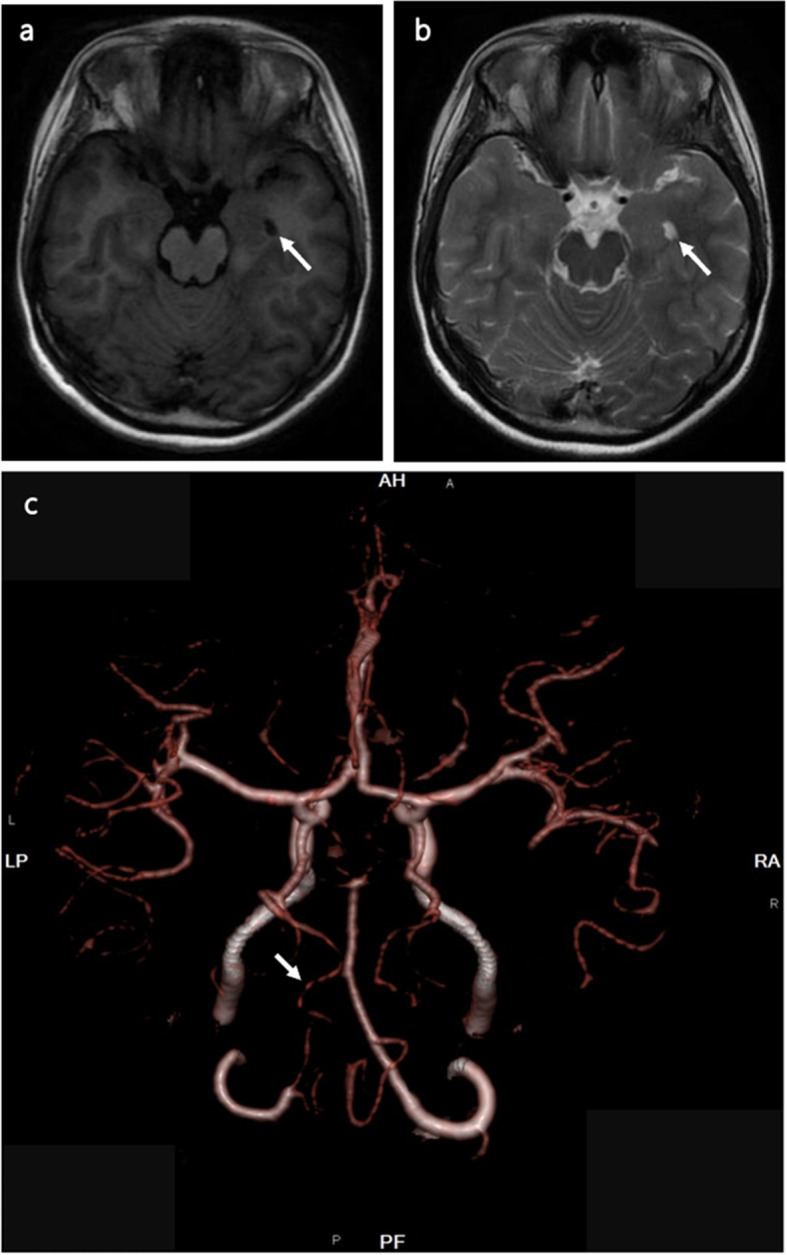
Brain magnetic resonance imaging (MRI) of the patient. a Dilation of the left ventricle on axial T1-weighted imaging (T1-WI). b Dilation of the left ventricle on axial T2-WI. c Cerebral CTA examination revealed multiple calcifications in the bilateral vertebral arteries and the V4 segment of the left vertebral artery was significantly thinner
